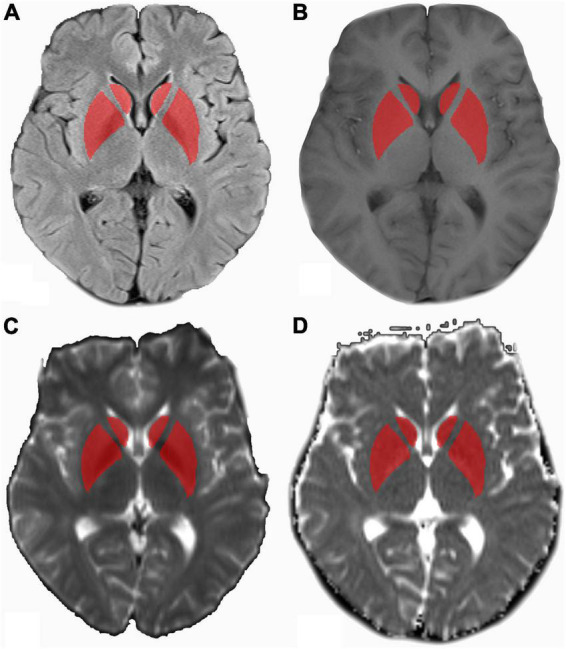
MR images in a 25-year-old patient with pre-eclampsia (PE). (A) Axial T2WI marked with ROI drawn on bilateral basal ganglia referring to axial T1WI (B), axial diffusion-weighted imaging (DWI) (*b* = 1,000 s/mm^2^) (C), and apparent diffusion coefficient (ADC) maps (D). ADC, apparent diffusion coefficient; DWI, diffusion-weighted imaging; T1WI, T1-weighted imaging; T2WI, T2-weighted imaging; ROI, region of inter.
